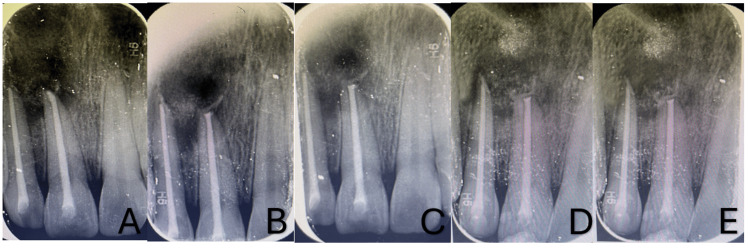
Follow-up radiographs A) baseline B) one week C) one month D) three months E) six months
